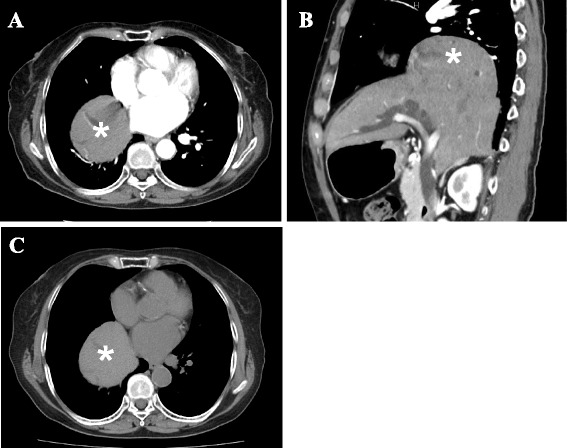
a, b Findings from preoperative thoracic and abdominal computed tomography (CT) for gastric cancer. Transverse CT shows the right hepatic lobe in the right inferior intrathoracic area (a: *asterisk*). On coronal section, the *asterisk* indicates the right hepatic lobe herniating into the right hemithorax via a diaphragmatic defect (b). c Thoracic and abdominal CT before radiotherapy for cervical cancer. The right hepatic lobe has herniated into the right hemithorax (*asterisk*), and appears no different in terms of the size of herniated liver compared to the preoperative CT for gastric cancer
